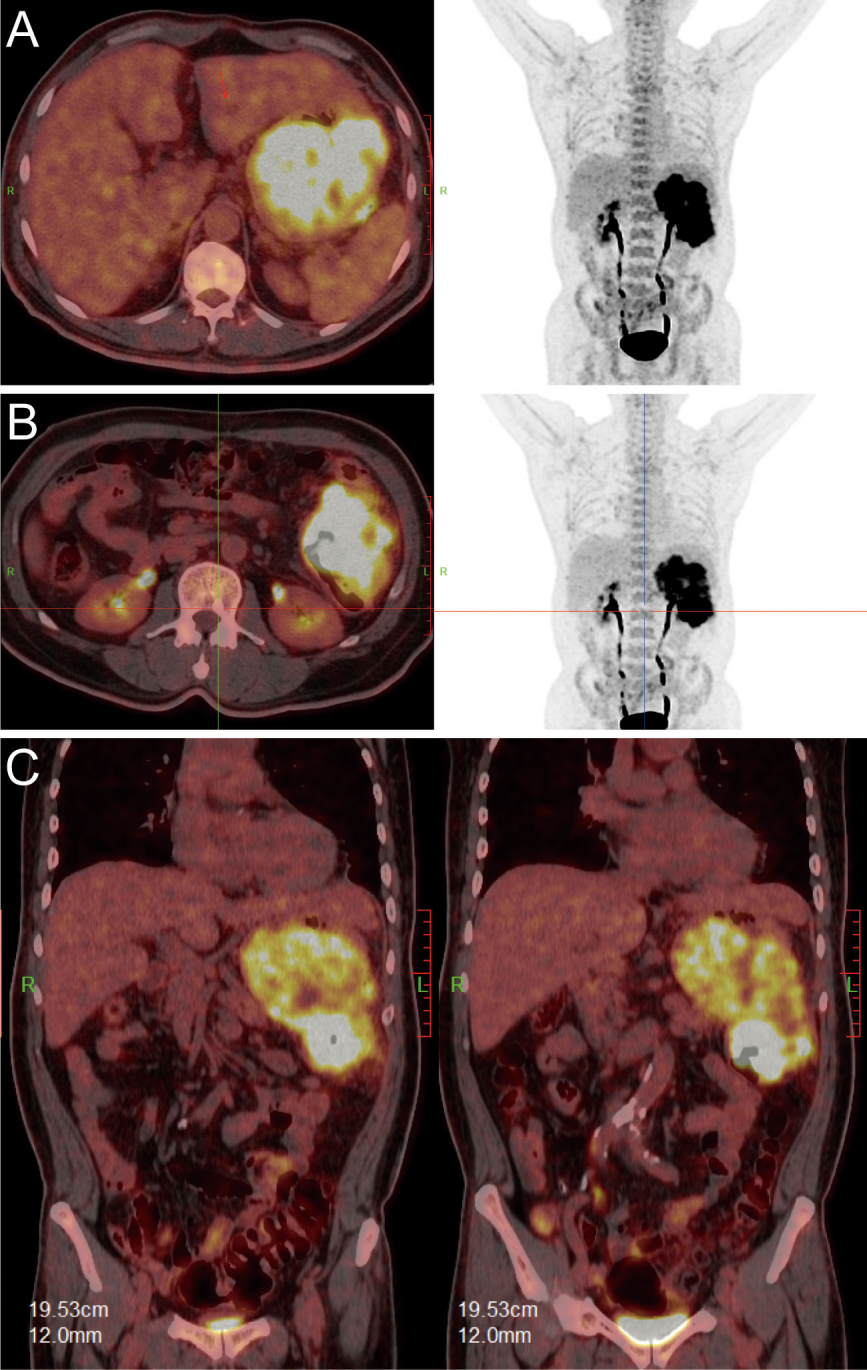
PET/CT fusion images. [(A, B): axial; (C): coronal] demonstrating hypermetabolic tumor involvement of the splenic flexure (SUVmax 39).
